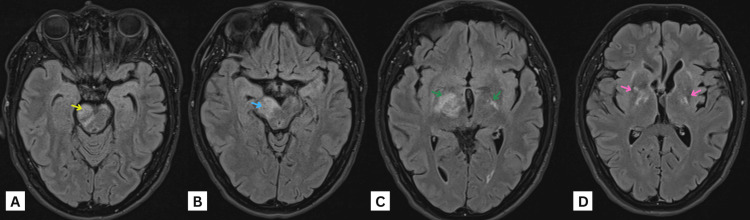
MRI of the brain axial FLAIR images at the level of the (A) pons, (B) midbrain, (C) upper midbrain, and (D) basal ganglia showing bilateral, almost symmetrical, signal alteration in the form of T2-FLAIR high-signal intensity involving the globus pallidus (pink arrows), corticospinal tracts (green arrows), and corona radiata. It starts from the corona radiata superiorly at the level of lateral ventricles extending to cerebral peduncles of the midbrain (blue arrows) inferiorly, affecting the right side more at this level. Similar signal alteration is also noted at the right anterolateral side of the pons (yellow arrows). No diffusion restriction can be seen. MRI: magnetic resonance imaging, FLAIR: fluid-attenuated inversion recovery
